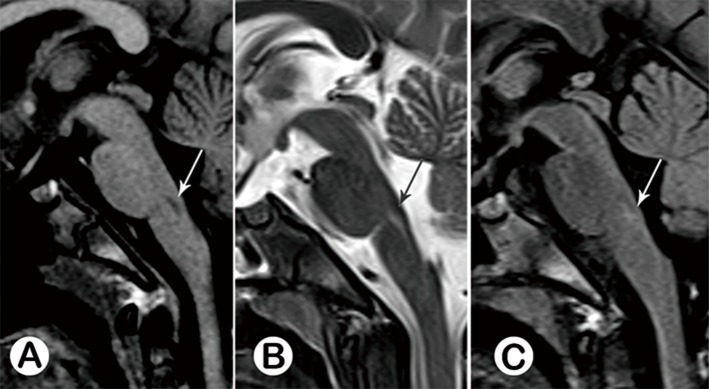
Follow-up study of previous case (Female, 6 years 8 months old,from Fig 2) in 3 years 8 months. Sagittal MR images revealed the lesion was smaller while compared with Fig 2, demonstrating prolonged T1 (A, white arrow) and prolonged T2 (B, black arrow) signal, and hyper-intensive signal on FLAIR(C, white arrow). This subject had special neurological sequelae, which were irregular breath and involuntary extremity tremor during sleeping at night.
